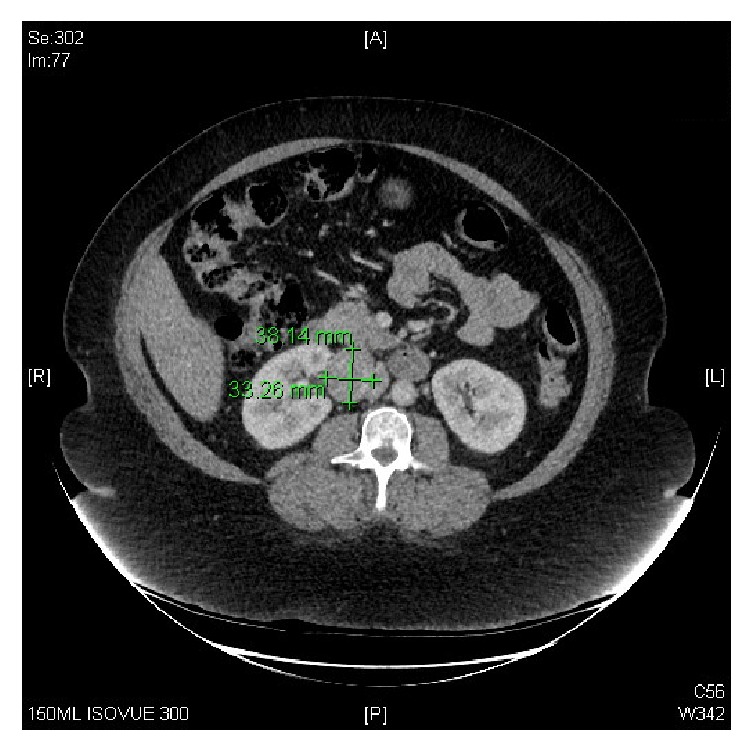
CT abdomen/pelvis with contrast.
